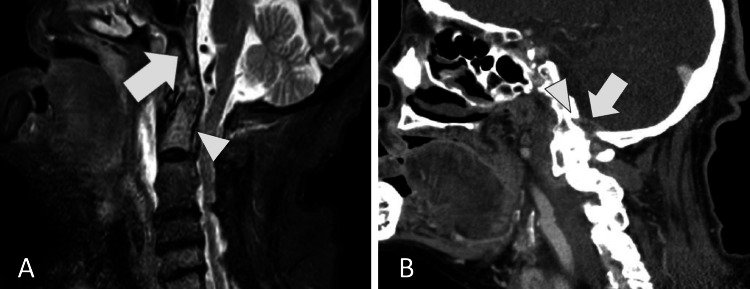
(A) Cervical spine MRI (STIR) with high signal intensity in the odontoid process (arrowhead) and the clivus (arrow). (B) Cervical spine contrast-enhanced CT with abscess (arrow) in the hypoglossal canal (arrowhead). STIR: Short Tau Inversion Recovery.
